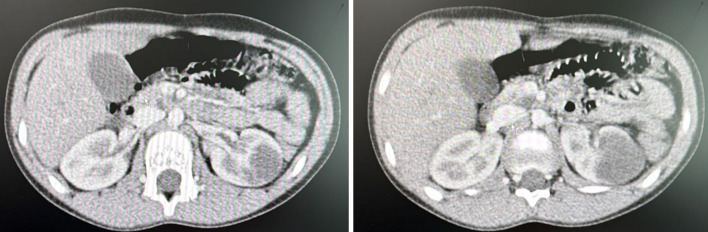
CT showing a left kidney mass (arrow). CT: computed tomography.
